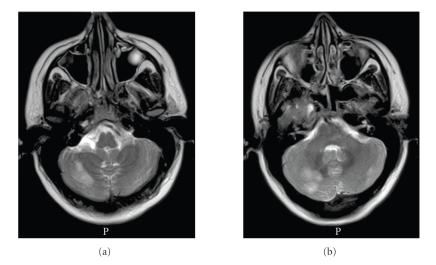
Axial T2-weighted MRI shows areas of hyperintense signal in both the right and left cerebellar hemispheres. Findings in this MRI had led to the initial false diagnosis of an ischemic stroke in the posterior circulation.
